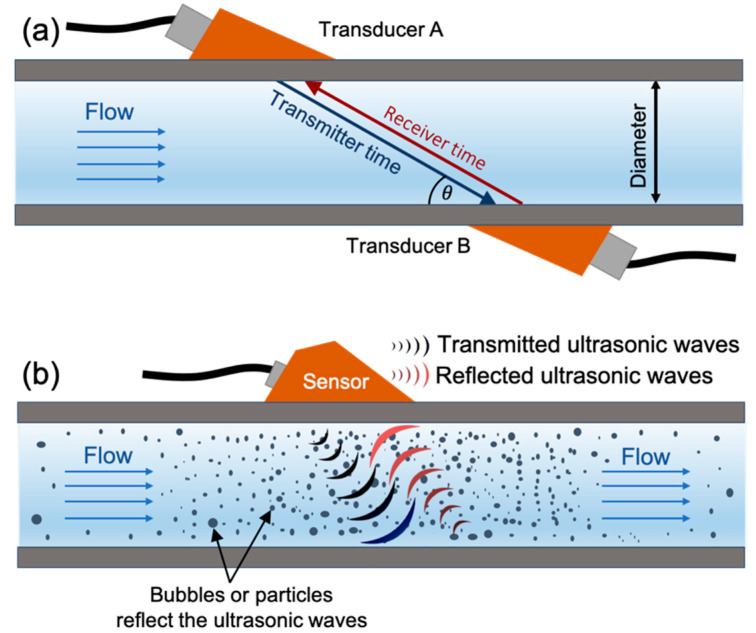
Different ultrasonic flowmeters types. (a) Transit time ultrasonic flowmeter. The fluid flow velocity depends on the tube diameter, the transmitter time for ultrasonic wave travels from transducer A to B, the receiver time, and the angle between the transducer and pipe θ. (b) Doppler ultrasonic flowmeter serves fluids with suspended bubbles or particles to reflect the transmitted ultrasonic wave.
